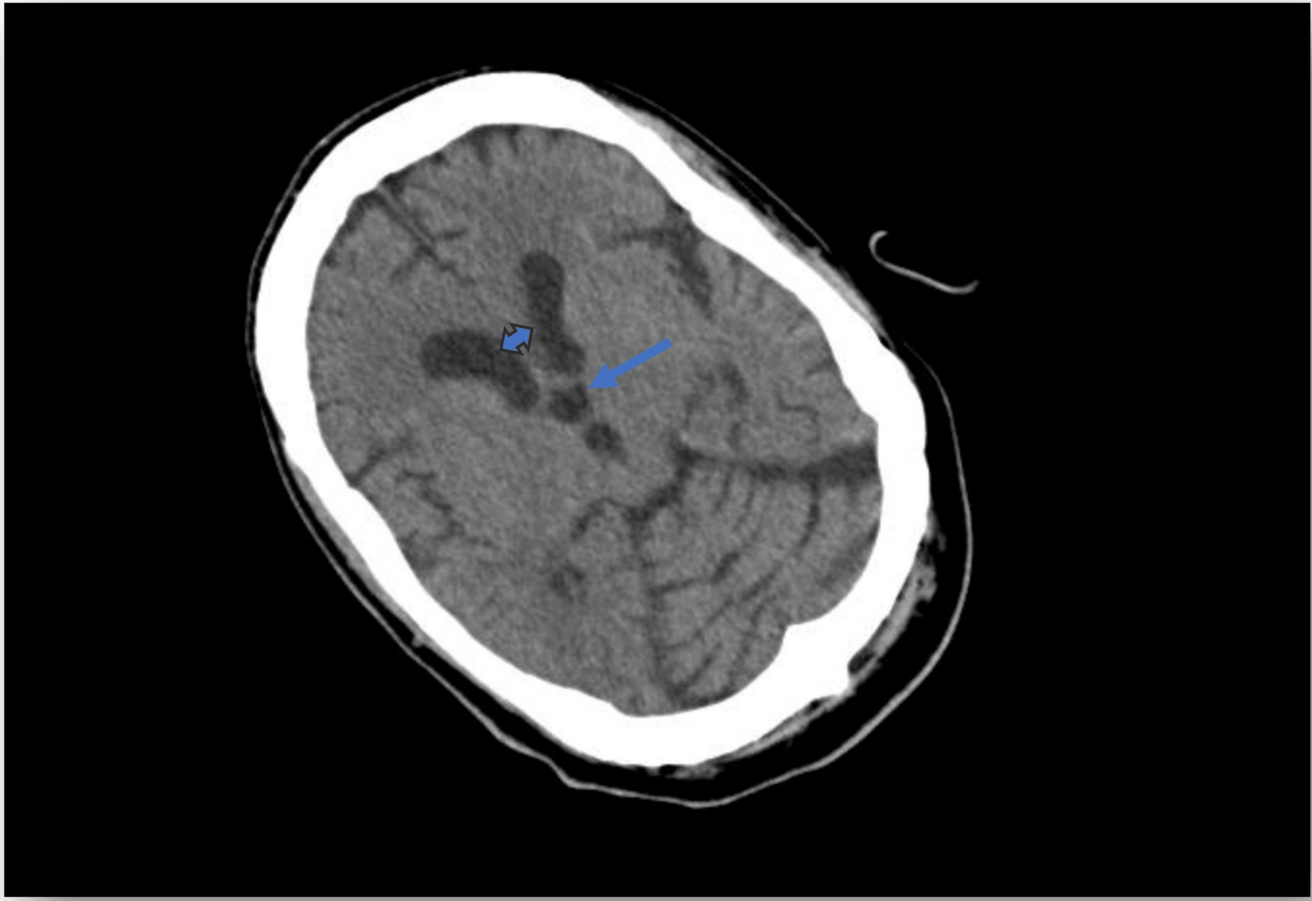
CT brain scan (axial view) of the patient. Note the dilatation of the third ventricle (dashed arrow) and the increased distance between the bodies of the lateral ventricles (double-headed arrow).
